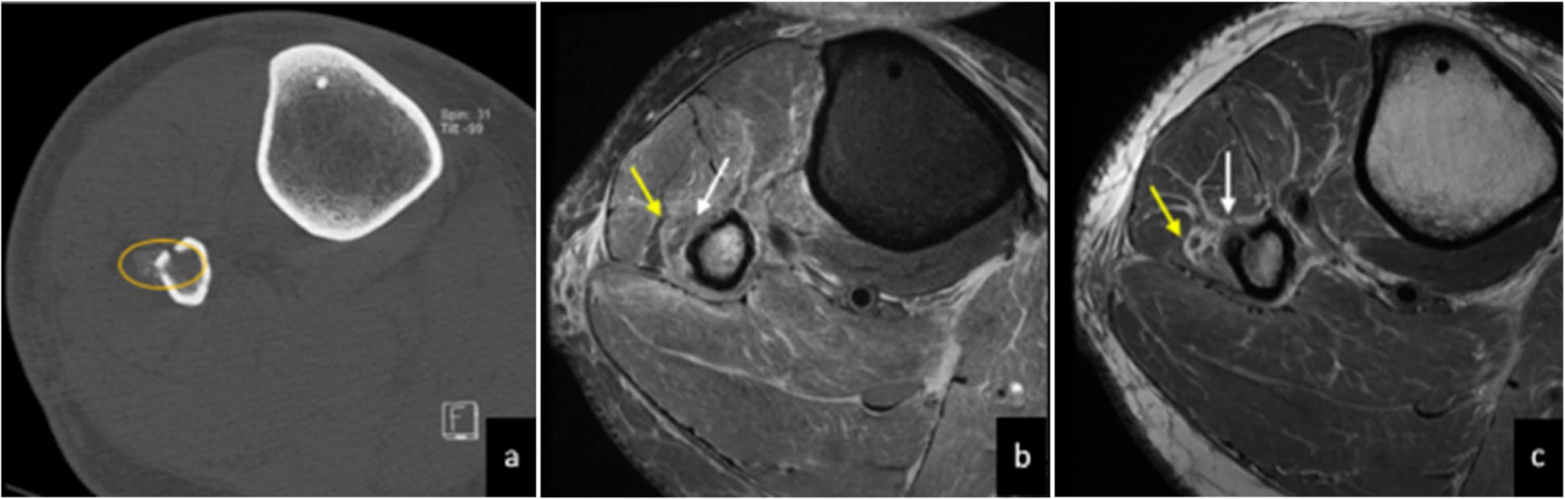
40-year-old man who had a skiing accident and sustained a high fibular fracture and syndesmotic injury. Soon after the injury, he presented with foot drop and symptoms of common peroneal nerve entrapment. CT at the level of the proximal fibula/calf shows periosteal new bone formation at site of the proximal fibular fracture (a). Sequential axial PDFS (b) and axial PD (c and d) sequences show the proximal superficial nerve (yellow arrows) and deep peroneal nerve (white arrows) shortly after the common peroneal nerve division. The deep peroneal nerve appears oedematous on the PDFS axial sequence. The superficial peroneal nerve appears oedematous, although to a lesser extent
